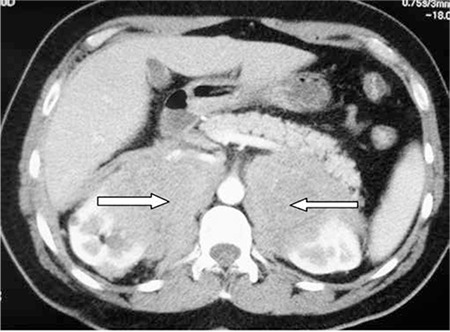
Postcontrast CT scan showed bilateral adrenal tumors (⇒).
